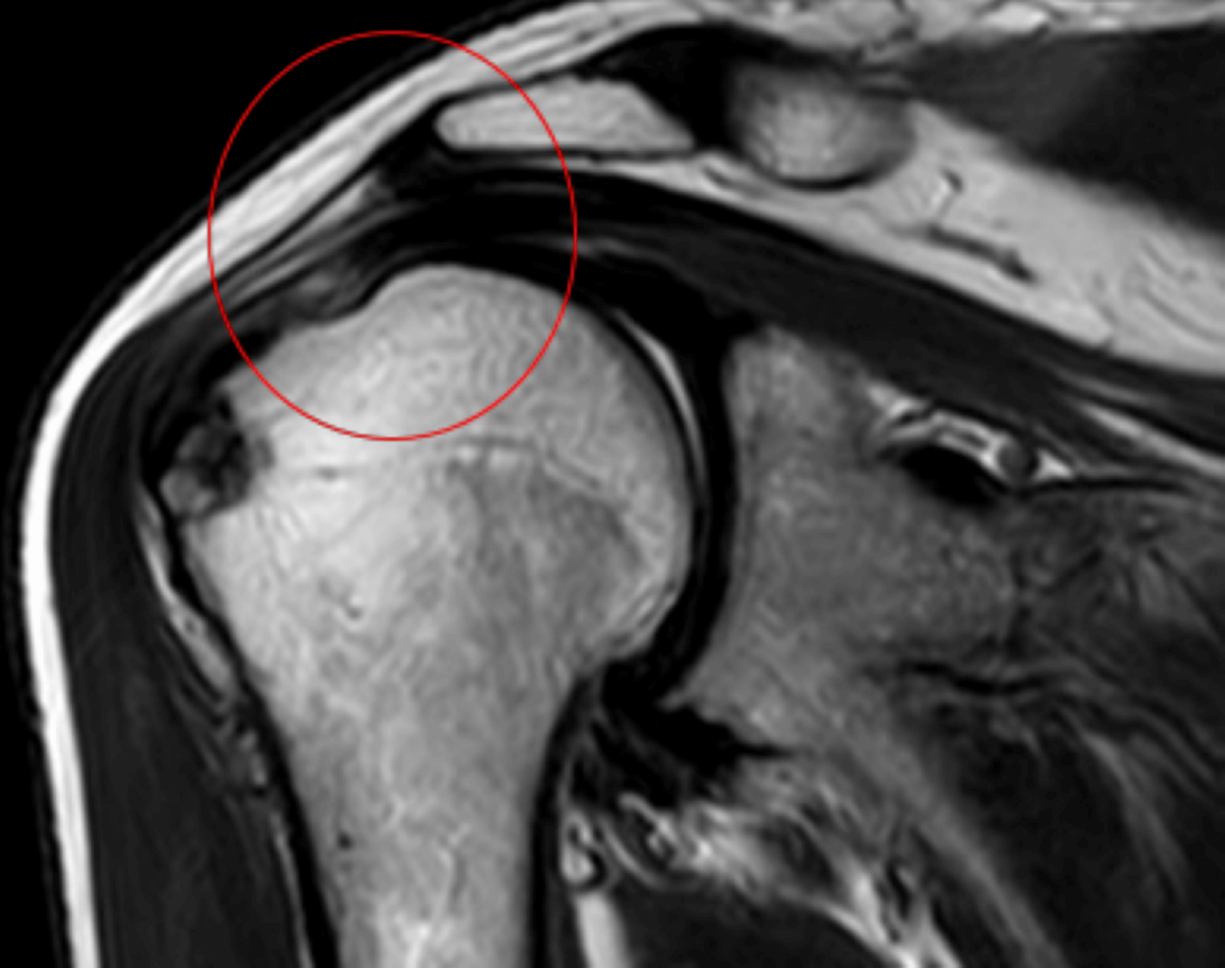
MRI of the right shoulder joint signifies partial tear of the supraspinatus muscle MRI: Magnetic resonance imaging The circle represents a partial tear of the supraspinatus muscle
